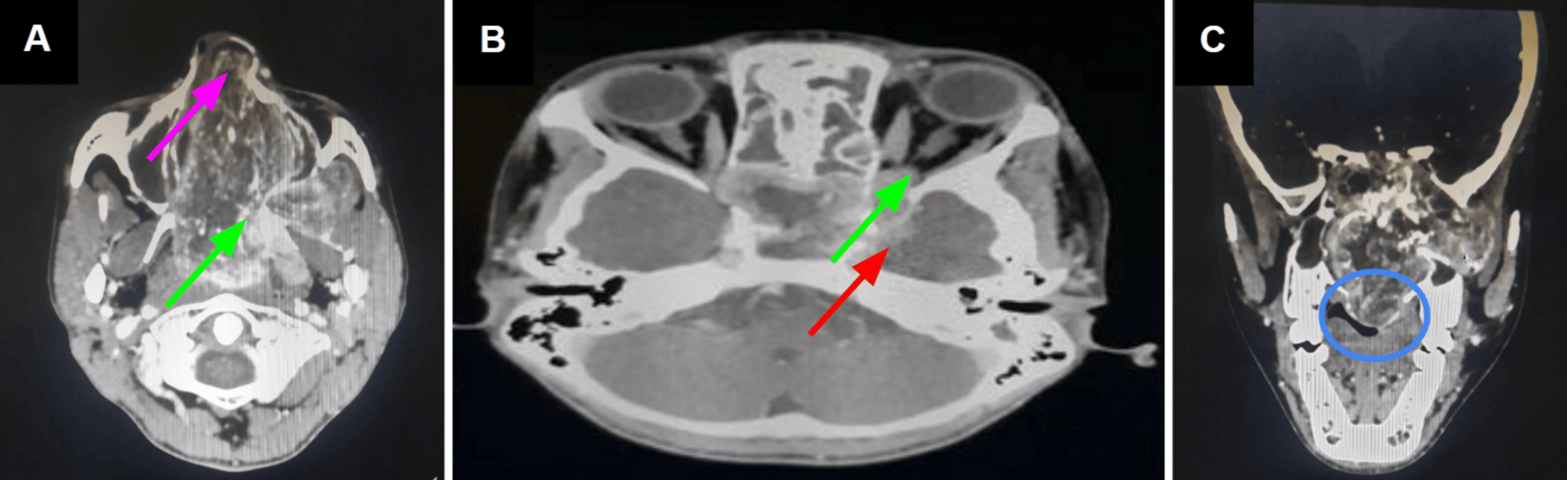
Contrast enhanced computed tomography axial (A & B) and coronal (C) images. (A) An avidly enhancing mass is noted in the nasopharyngeal region, centered in the left sphenopalatine foramen, causing its widening (green arrow) and extending towards the right side. The main bulk is noted in the left nasal cavity (pink arrow). (B) Intraorbital (green arrow) and intracranial (red arrow) extension into the left middle cranial fossa was also noted, as evident by the enhancing component. (C) It is extending into adjacent soft tissues and also causing erosion of bony walls. Invasion of the paranasal sinuses was also evident, with involvement of the hard palate (blue circle).
